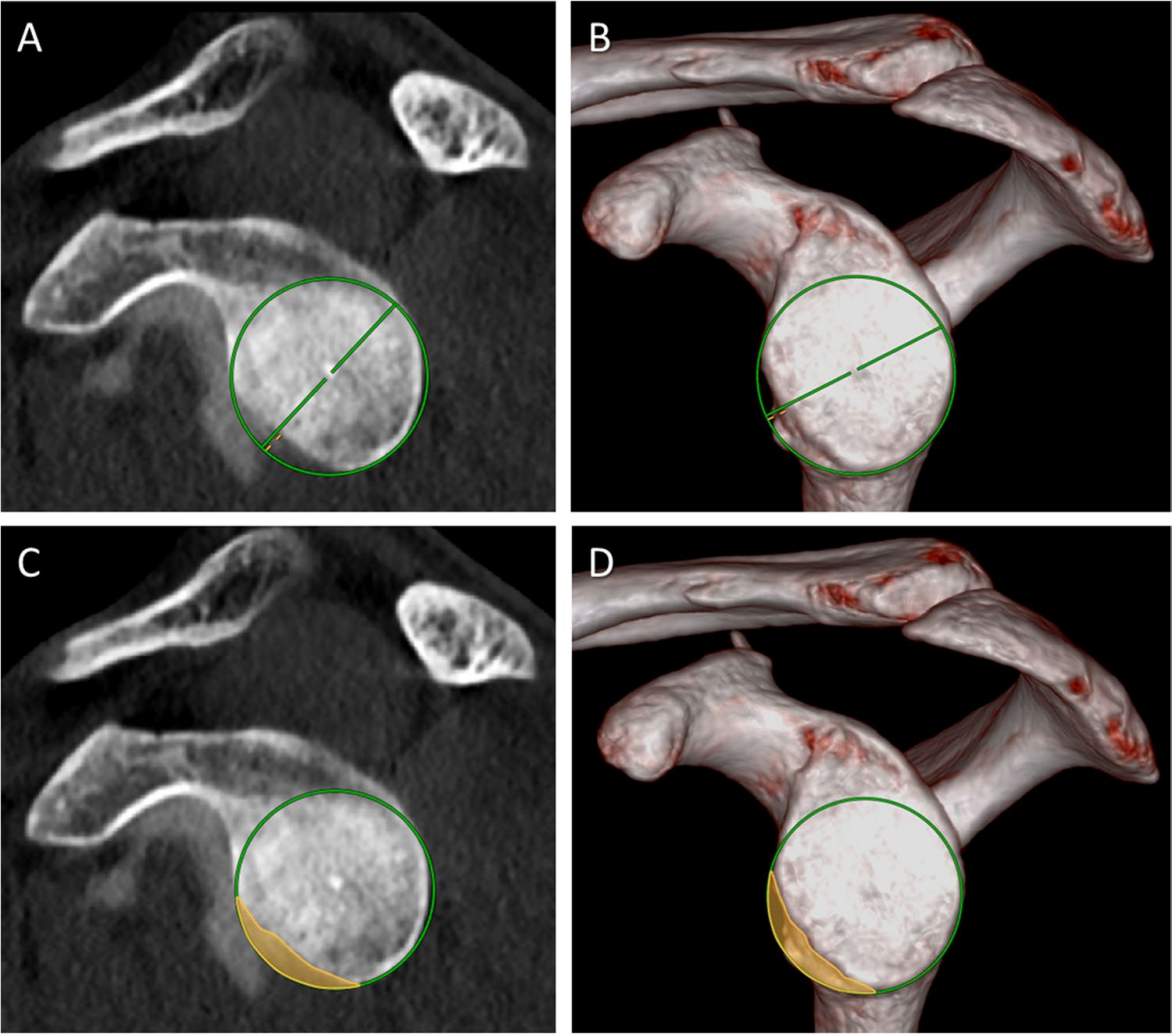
A 28-year-old male with bony Bankart lesion of the anteroinferior glenoid. Dual energy CT after arthrography of the right shoulder with reformatted sagittal en face 2D arthrogram images (A and C) and en face 3D VNC VRT reformats of the glenoid (B and D). Measurement of the glenoid diameter (green lines) using the best fit circle method was comparable with 30.9 mm on the 2D image (A) and 31.4 mm on the 3D image (B). The width of the glenoid defect was measured identical with 3.1 mm (yellow lines in (A) and (B)). The glenoid surface area (green circles) was also measured similar on the 2D image (C) and 3D image (D) with 751 mm2 and 775 mm2, respectively. Similarly, the surface area of the osseous glenoid defect (yellow areas) was measured with 49 mm2 on image (C) and 44 mm2 on image (D)
